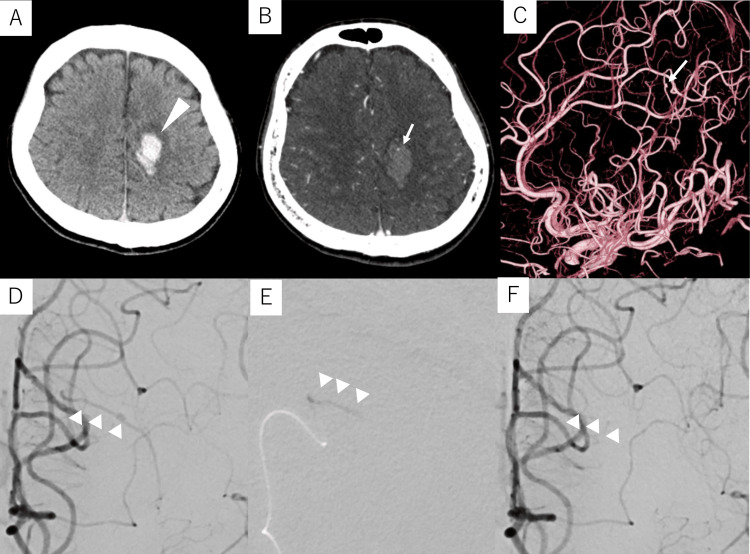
Preoperative and intraoperative imaging of Case 1 Case 1: A 38-year-old male with intracerebral hemorrhage (A) Computed tomography (CT) upon admission shows a left frontal subcortical hemorrhage (arrowhead). (B) Contrast-enhanced CT shows an enhanced spot (arrow) within the hematoma. (C, D) Digital subtraction angiography (DSA) of the left internal carotid artery shows a 1.1-mm fusiform aneurysm (arrow) on a 0.5-mm peripheral branch (arrowheads) of the left anterior cerebral artery. (E) The aneurysm and the parent artery are embolized with 33% N-butyl cyanoacrylate glue (arrowheads). (F) Post-embolization DSA of the left internal carotid artery shows the disappearance of the aneurysm (arrowheads).
